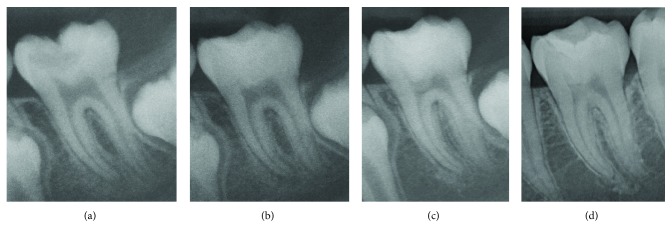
Periapical radiographs recorded (a) before treatment, (b) immediately after treatment, (c) six months postoperative, and (d) five years postoperative, showing dentine bridge and improvement of the periapical area.
